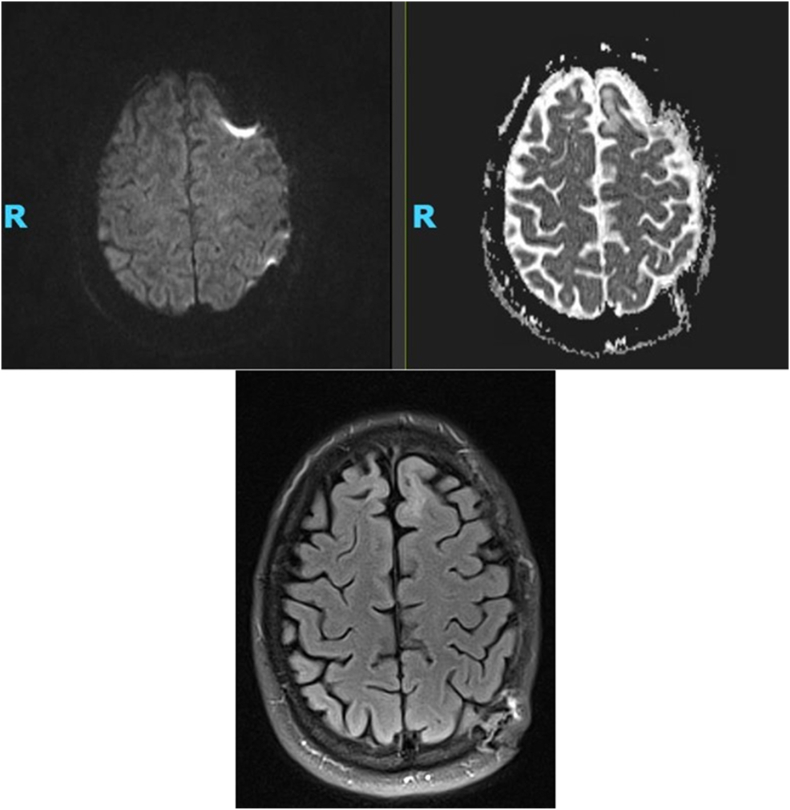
Left: DWI sequence. Right: ADC sequence. Bottom: T2-weighted FLAIR image. Resolution of hyperintensities on DWI with no new diffusion restriction, indicating no acute intracranial ischemic infarct. No pseudonormalization of the ADC sequence and no residual FLAIR hyperintensities, consistent with complete reversal of ischemic MRI changes. Follow-up images were acquired on a 3T MRI scanner. DWI acquisitions were trace-based with 3-mm slice thickness and 0.4-mm interslice gap. ADC scans were performed with identical parameters. T2-FLAIR images were acquired with a 3-mm slice thickness and 0.4-mm interslice gap. Quantification of DWI signal changes and ADC values is not feasible due to differences in MRI acquisition parameters and scanner calibration across studies, which precludes longitudinal quantification.
